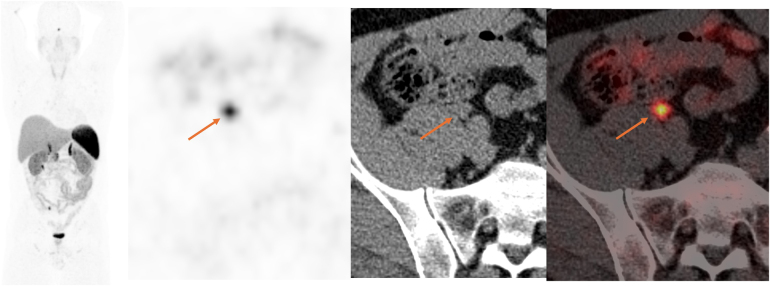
68Ga-DOTATATE maximal intensity image, positron emission tomography (PET), computed tomography (CT) and PET/CT demonstrates intense uptake in the lesion anterior to the right psoas muscle (arrows), Krenning score 3.
